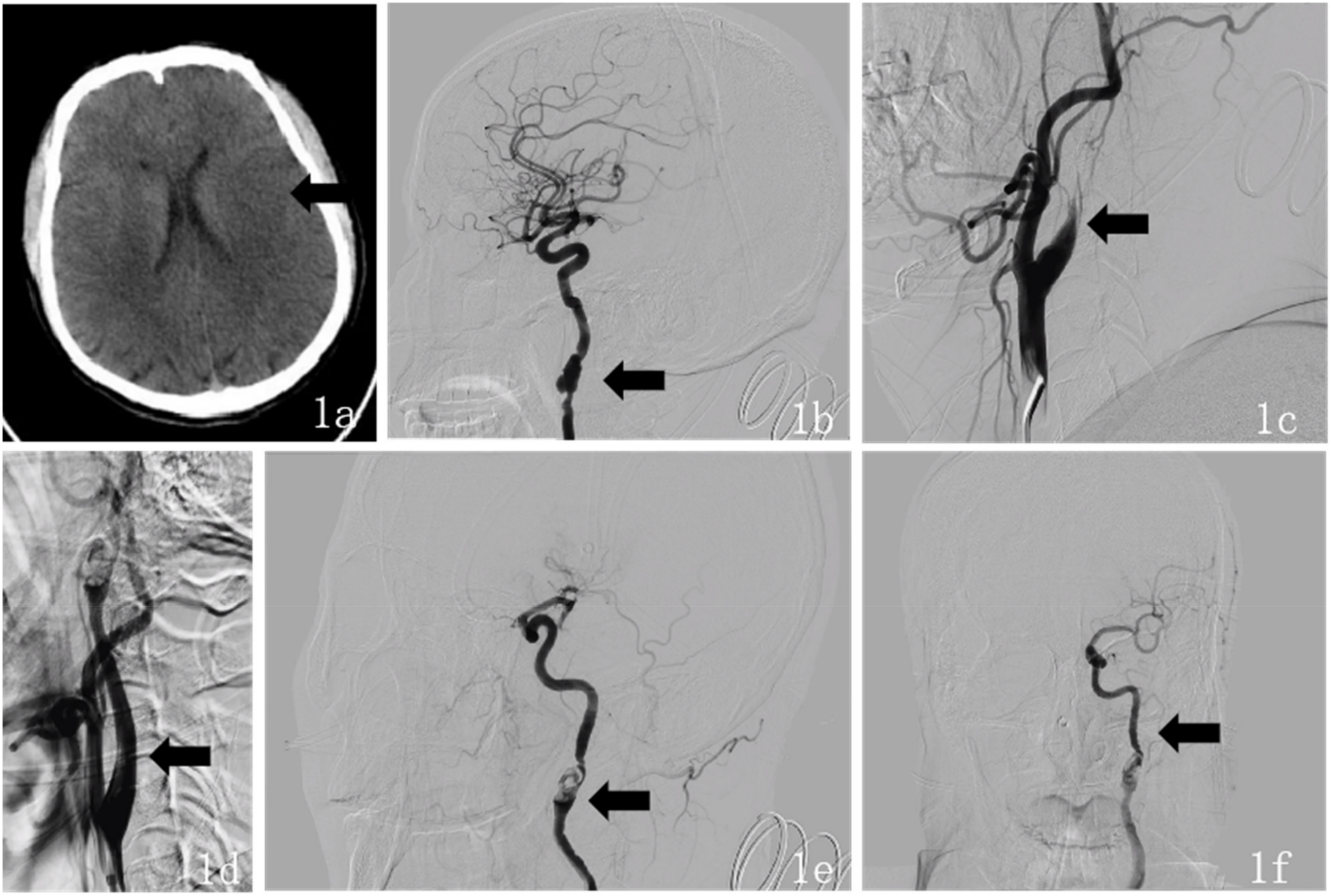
(a) Preoperative (October 17, 2024) head CT findings: axial CT shows a large area of slightly low-density lesions in the left temporal-parietal lobe. (b) Intraoperative DSA findings during endovascular therapy: The lateral DSA demonstrates a “string of beads” appearance in the right ICA, accompanied by a dissecting aneurysm in the C1 segment. (c) Lateral DSA shows occlusion at the origin of the left ICA. (d) Intraoperative lateral DSA shows recanalization of the left ICA. (e) Intraoperative lateral DSA revealed areas of alternating stenosis and dilatation in the left ICA, with a dissecting aneurysm in the C1 segment. (f) Intraoperative anteroposterior DSA shows “string of beads” changes in the left ICA at the C1 segment, with restored distal blood flow.
